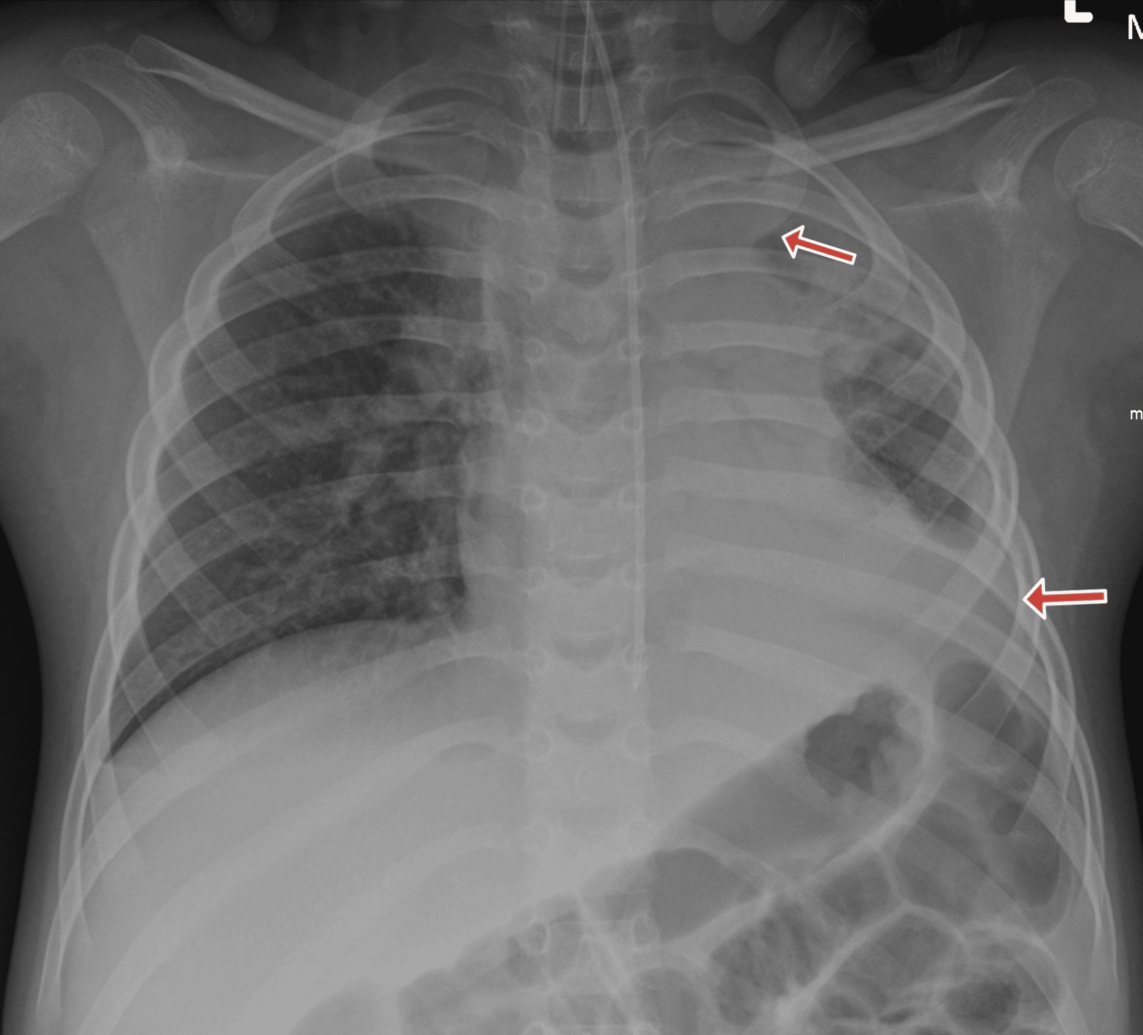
Chest X-ray showing consolidations in both upper lobes, more marked on the left (highlighted with red arrows).
